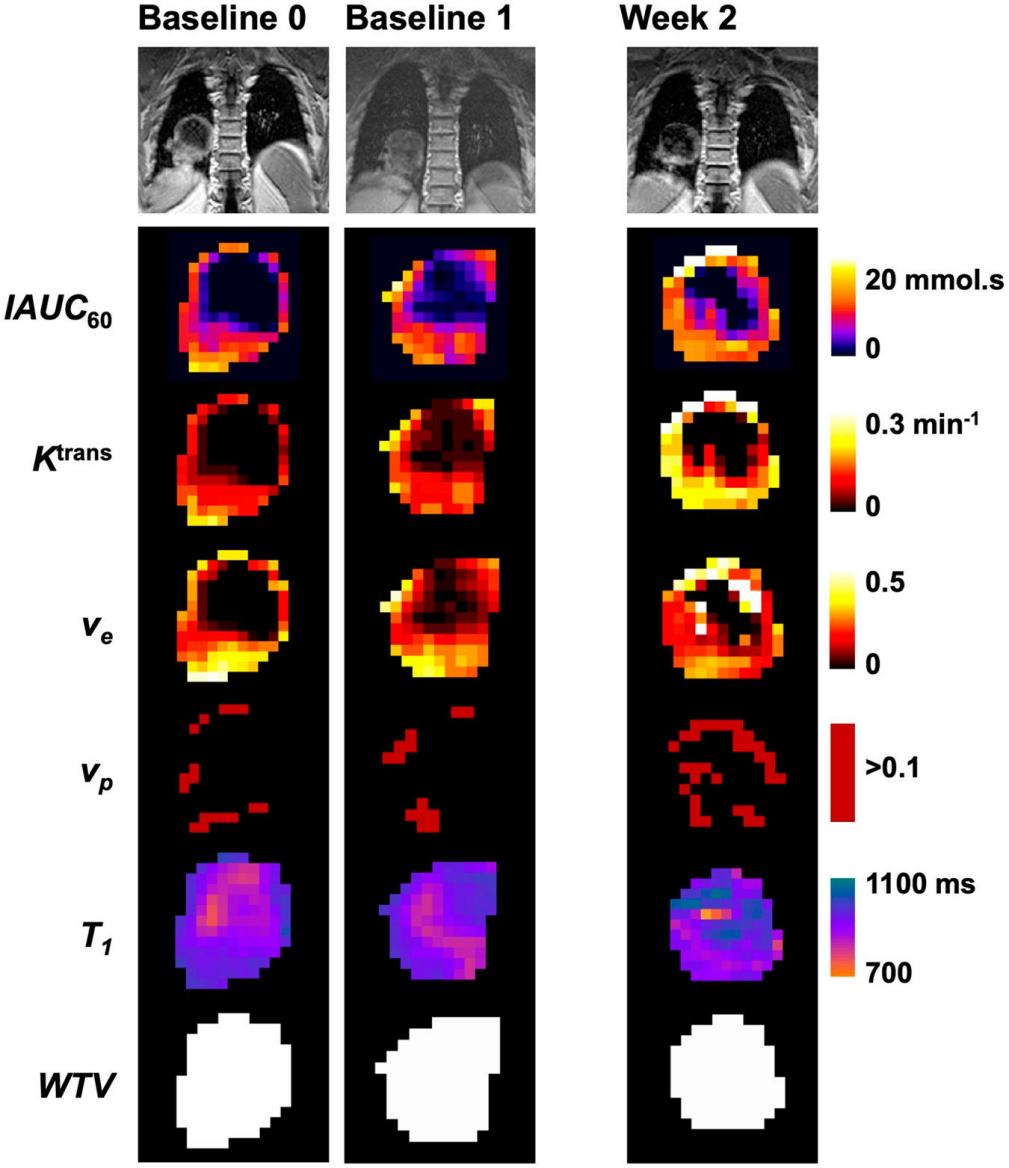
Example images of primary lung cancer in a patient who underwent MRI on all three occasions
